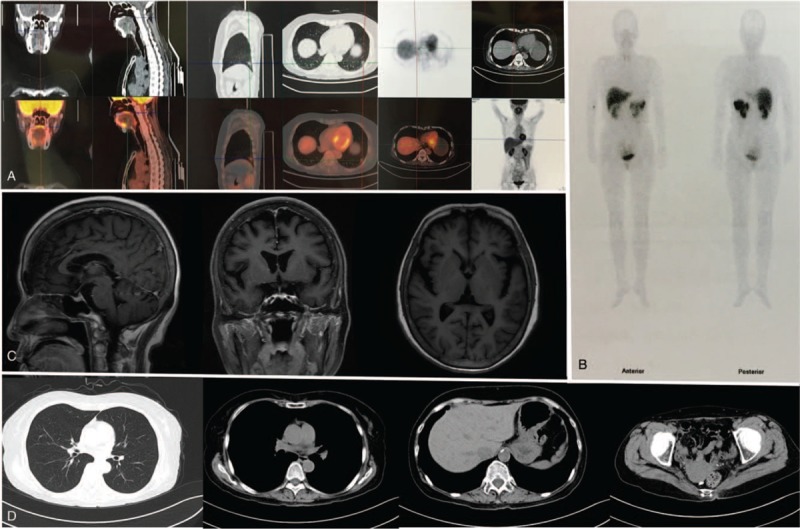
Imaging materials of the patient. (A) ^18^F-PET/CT. (B) ^99m^Tc-HTOC-SPETCT. (C) Magnetic resonance imaging of craniocerebral. (D) Computed tomography of chest, abdomen, and pelvis.
